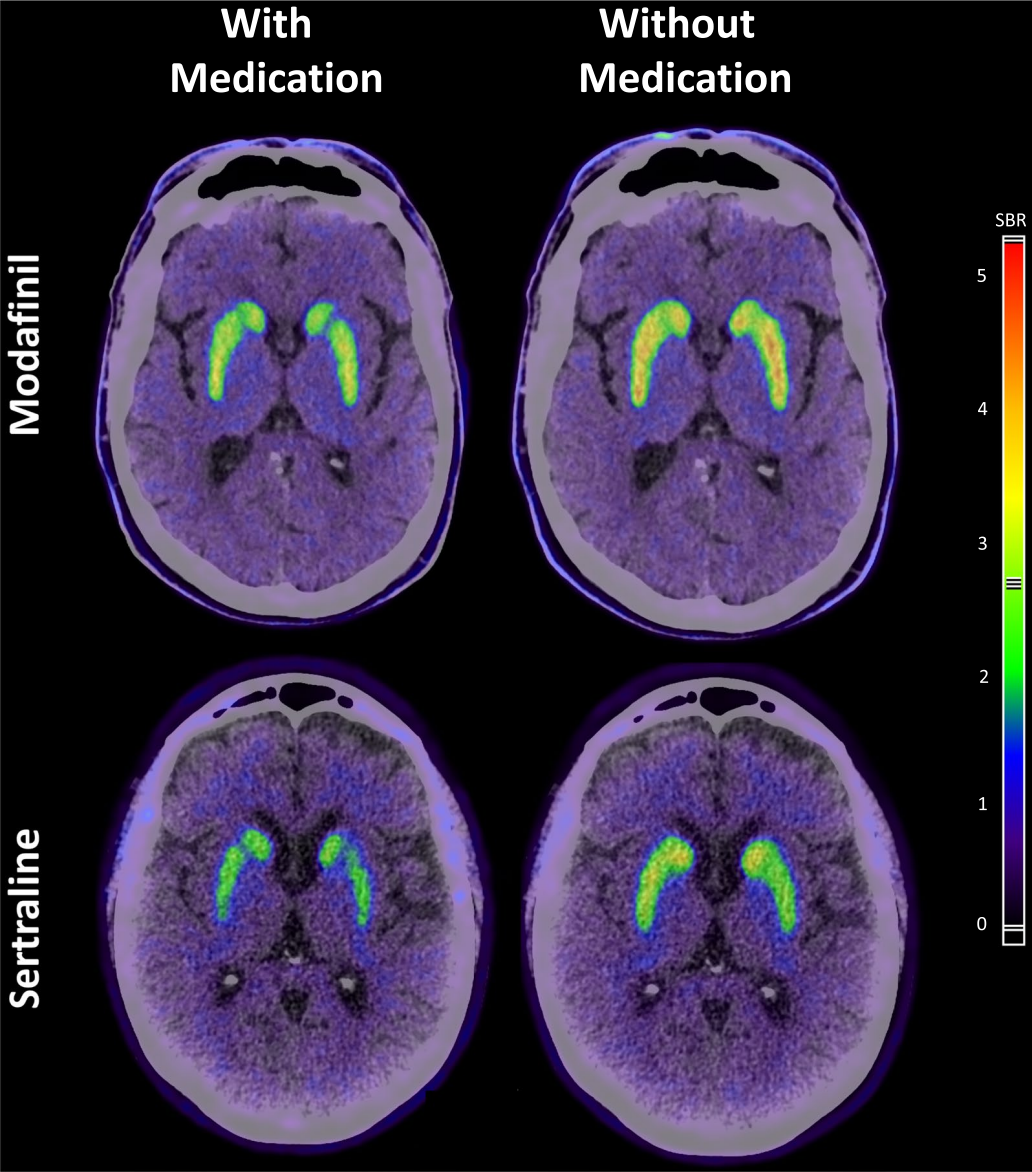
Axial sections of fused [^18^F]FE-PE2I PET and CT for visual comparison with and without 100-mg Modafinil (top) or 100-mg sertraline treatment (bottom) (patient 2)
